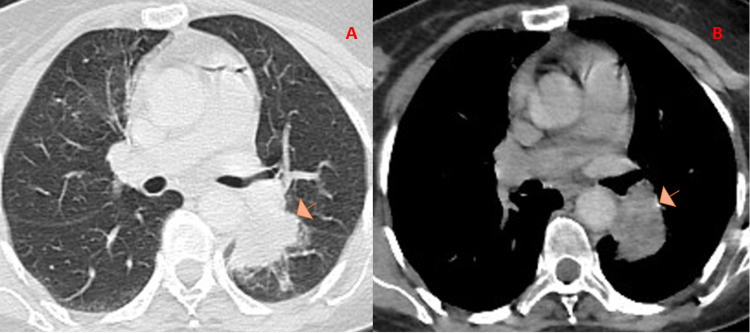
CT chest scan with IV contrast shows a suspected lesion of the left lobe. There is a heterogeneous enhancement after injection of the contrast product. It measured 50 mm on the long axis and 33 mm on the short axis. (A) Lung parenchyma window and (B) bone window CT chest.
